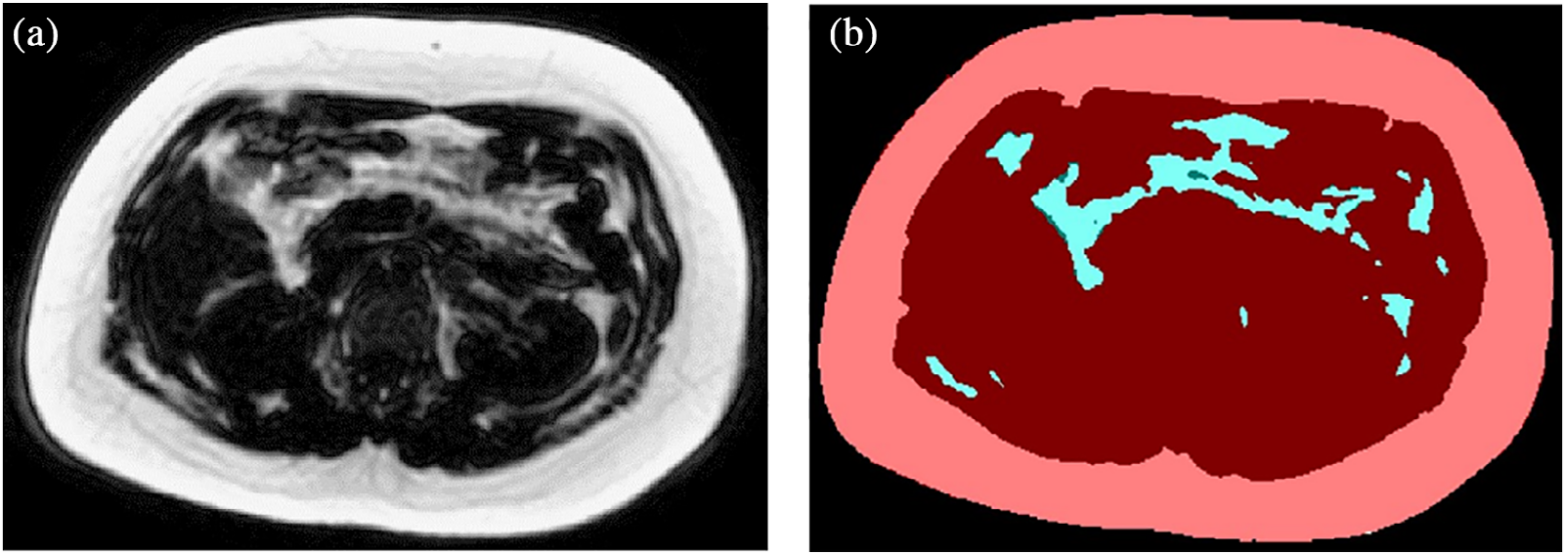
A, The original mDixon MRI fat fraction abdominal image of a single slice obtained at the level of lumbar 3 vertebrae. B, The extracted image from A, showing the subcutaneous adipose tissue (SAT) in colour pink and visceral adipose tissue (VAT) in colour blue using our validated in house method^31^
